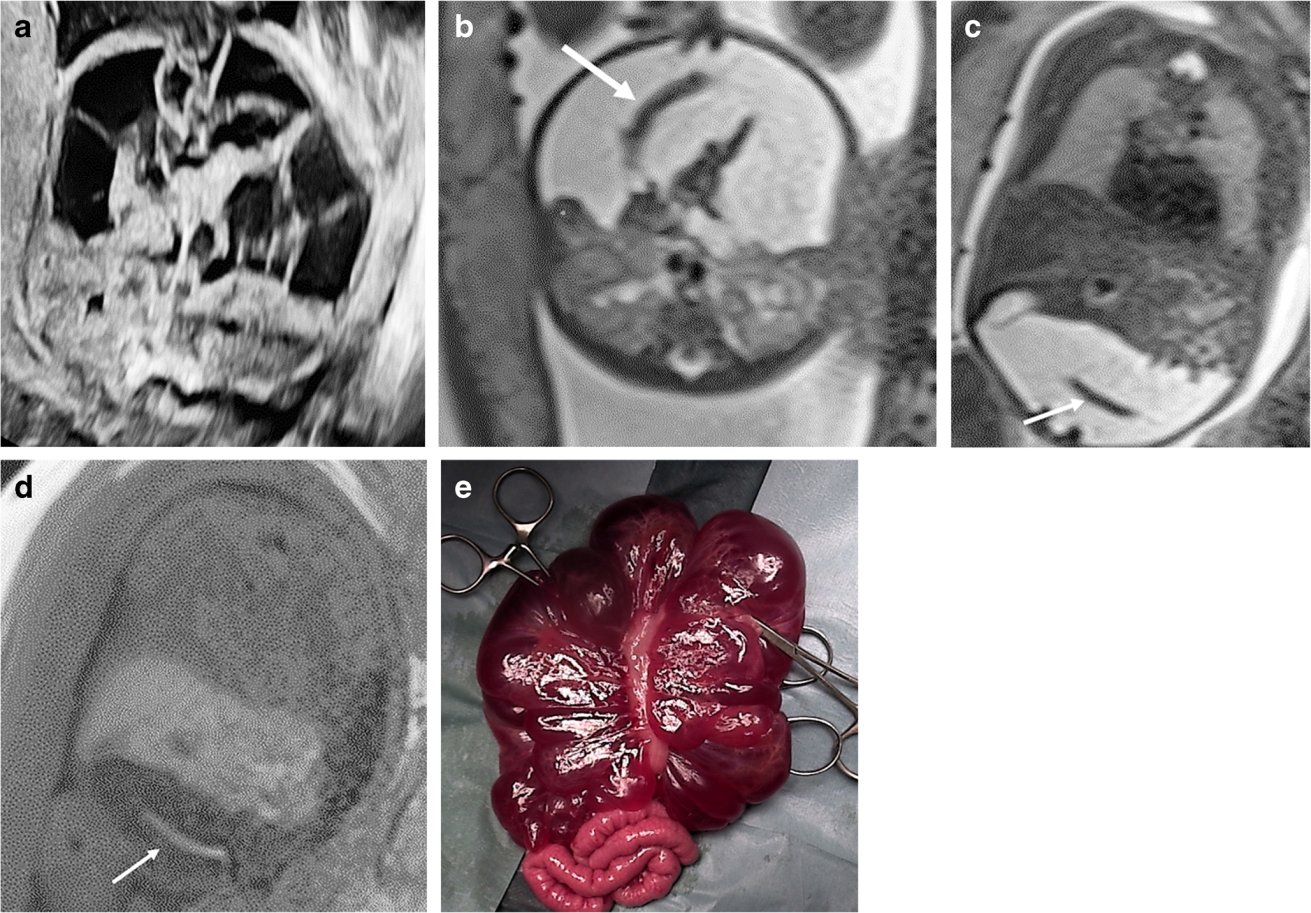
A male fetus at 35 weeks’ gestation with extensive mesenteric malformation and postnatal surgery at 4 months old (N18, Table 3). a–d Axial US (a) and T2-weighted MR (b) images (repetition time [TR]/echo time [TE] 1,200/90 ms) at the same level and coronal T2- (c) (TR/TE 1,200/90 ms) and T1-weighted (d) (TR/TE 3.29/1.29 ms) MR images show the extensive mesenteric macrocystic lymphatic malformation with homogenous liquid echogenicity and signal intensity and multiple internal septations. The lesion displaces the fetal intraperitoneal organs but shows no significant complication. The fetal colon, surrounded by the lymphatic malformation, is easily identifiable by its meconium filling, hypointense on T2- and hyperintense on T1-weighted images (*arrows*). The lesion was correctly identified only at MRI. e Postnatal image during the surgical procedure shows the voluminous lesion surrounding the colon
